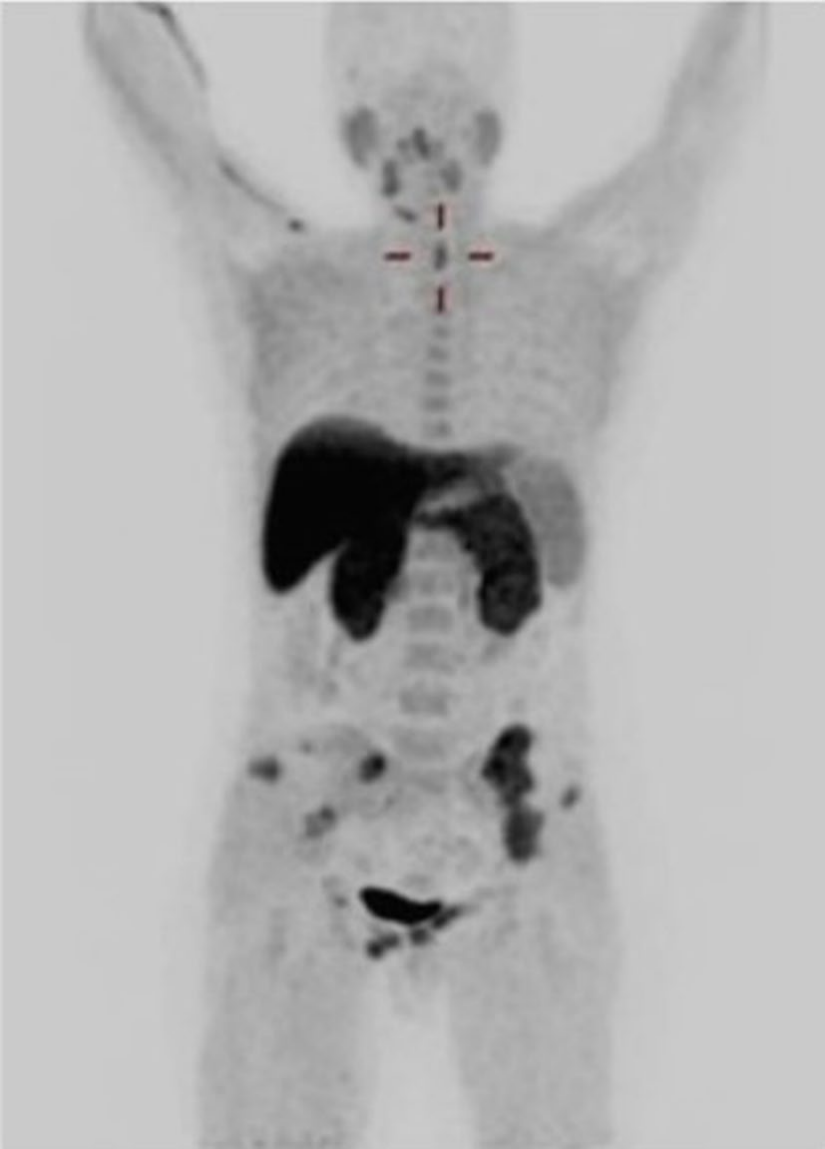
[^18^F]fluorocholine PET/CT maximum intensity projection
